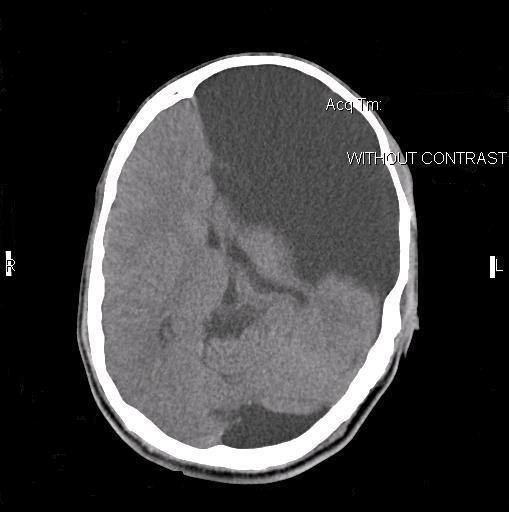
Postoperative brain CT scan of our patient after evacuation of the hematoma. Shows decompression of the cyst and underlying brain parenchyma.
